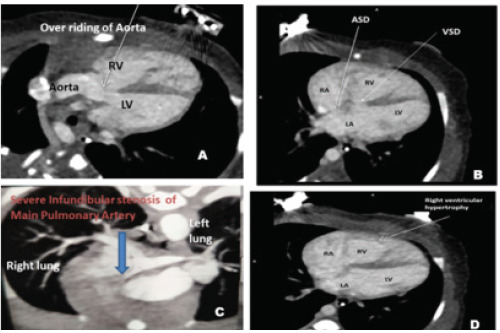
CT Angiogram showing overriding of aorta (A), Atrial septal defect and Ventricular Septal Defect (B), Severe infundibular stenosis of main pulmonary artery (C) and Right ventricular hypertrophy.
